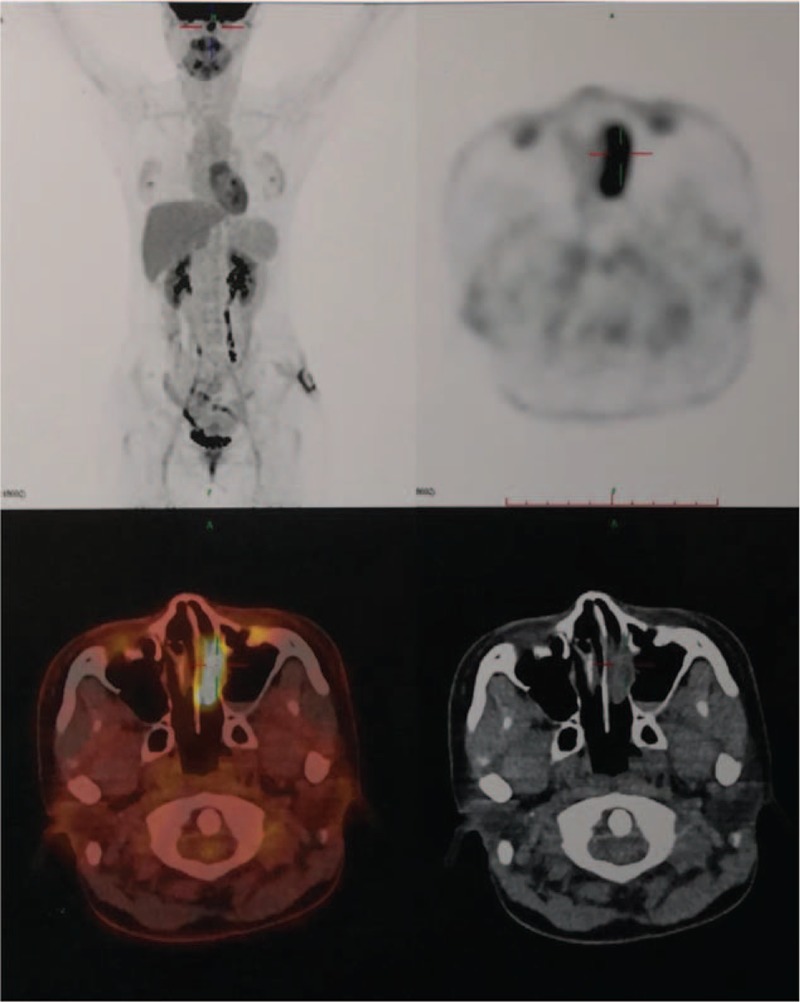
Positron emission tomography-computed tomography revealed abnormal high-intake in the left nasal cavity (1.2 × 3.3 × 1.4 cm, SUVmax 16.9).
